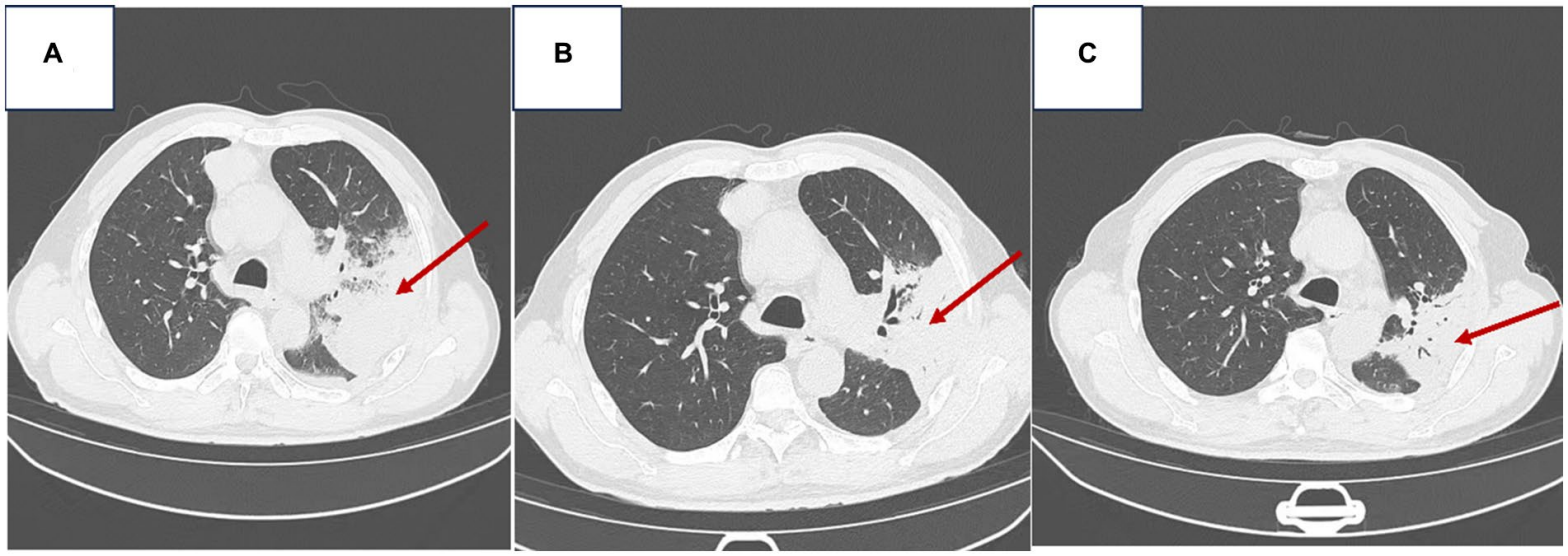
Chest imaging of the patient [(A) Admission chest CT on 07/26/2023; (B) Follow-up chest CT on 08/03/2023; (C) Follow-up chest CT on 08/10/2023].
